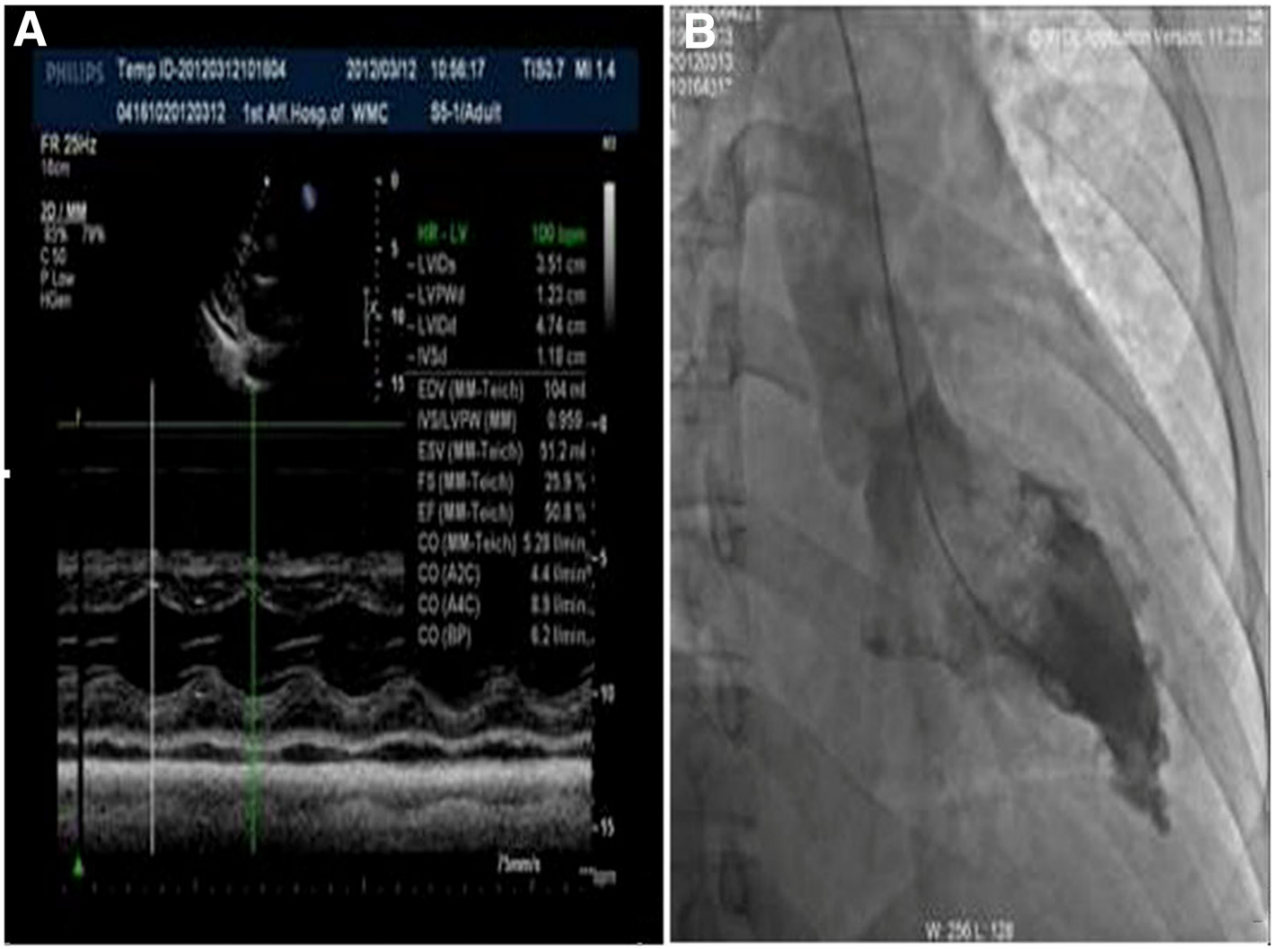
Echocardiography and left ventricular angiography. A. Echocardiography revealed normal movement of the ventricular wall and deteriorated diastolic function (left ventricular end diastolic diameter (LVEDD), 47 mm; interventricular septum thickness (IVS), 12 mm; left ventricular ejection fraction (LVEF), 50.8%). Coronary angiography showed that there was no stenosis or obstructive lesions in the coronary artery; B. Left ventricular angiography showed thickening of the apical ventricular membrane.
